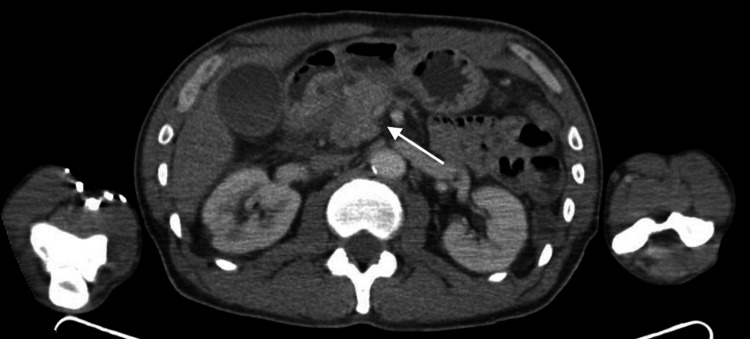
CT scan showing a hyperdense mass-like lesion in the pancreatic head and uncinate process (arrow) CT: computed tomography
